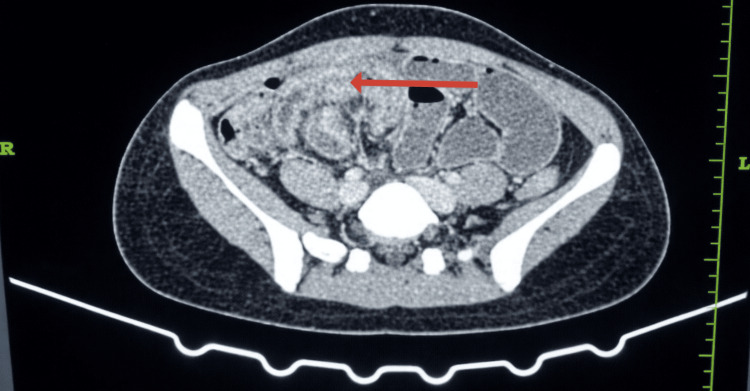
Enhancing membrane (cocoon) on CECT The dilated proximal bowel loops filled with gas are marked with an arrow. CECT: contrast-enhanced computed tomography
